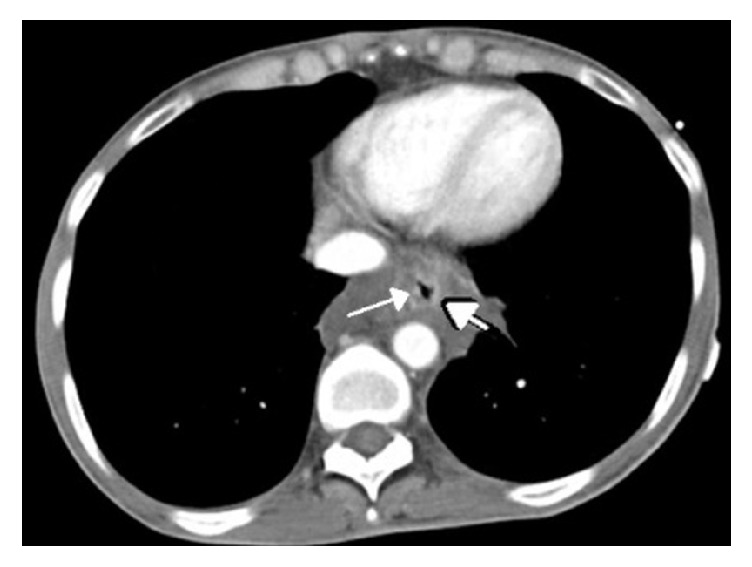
CT scan of the upper abdomen. Low-attenuation surrounding the distal esophagus with esophageal wall thickening (arrows).
